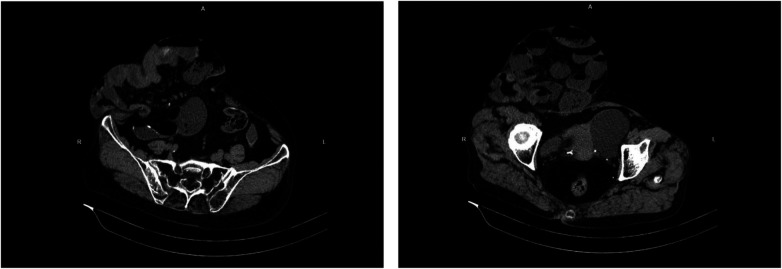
Preoperative CT image of the patient.
